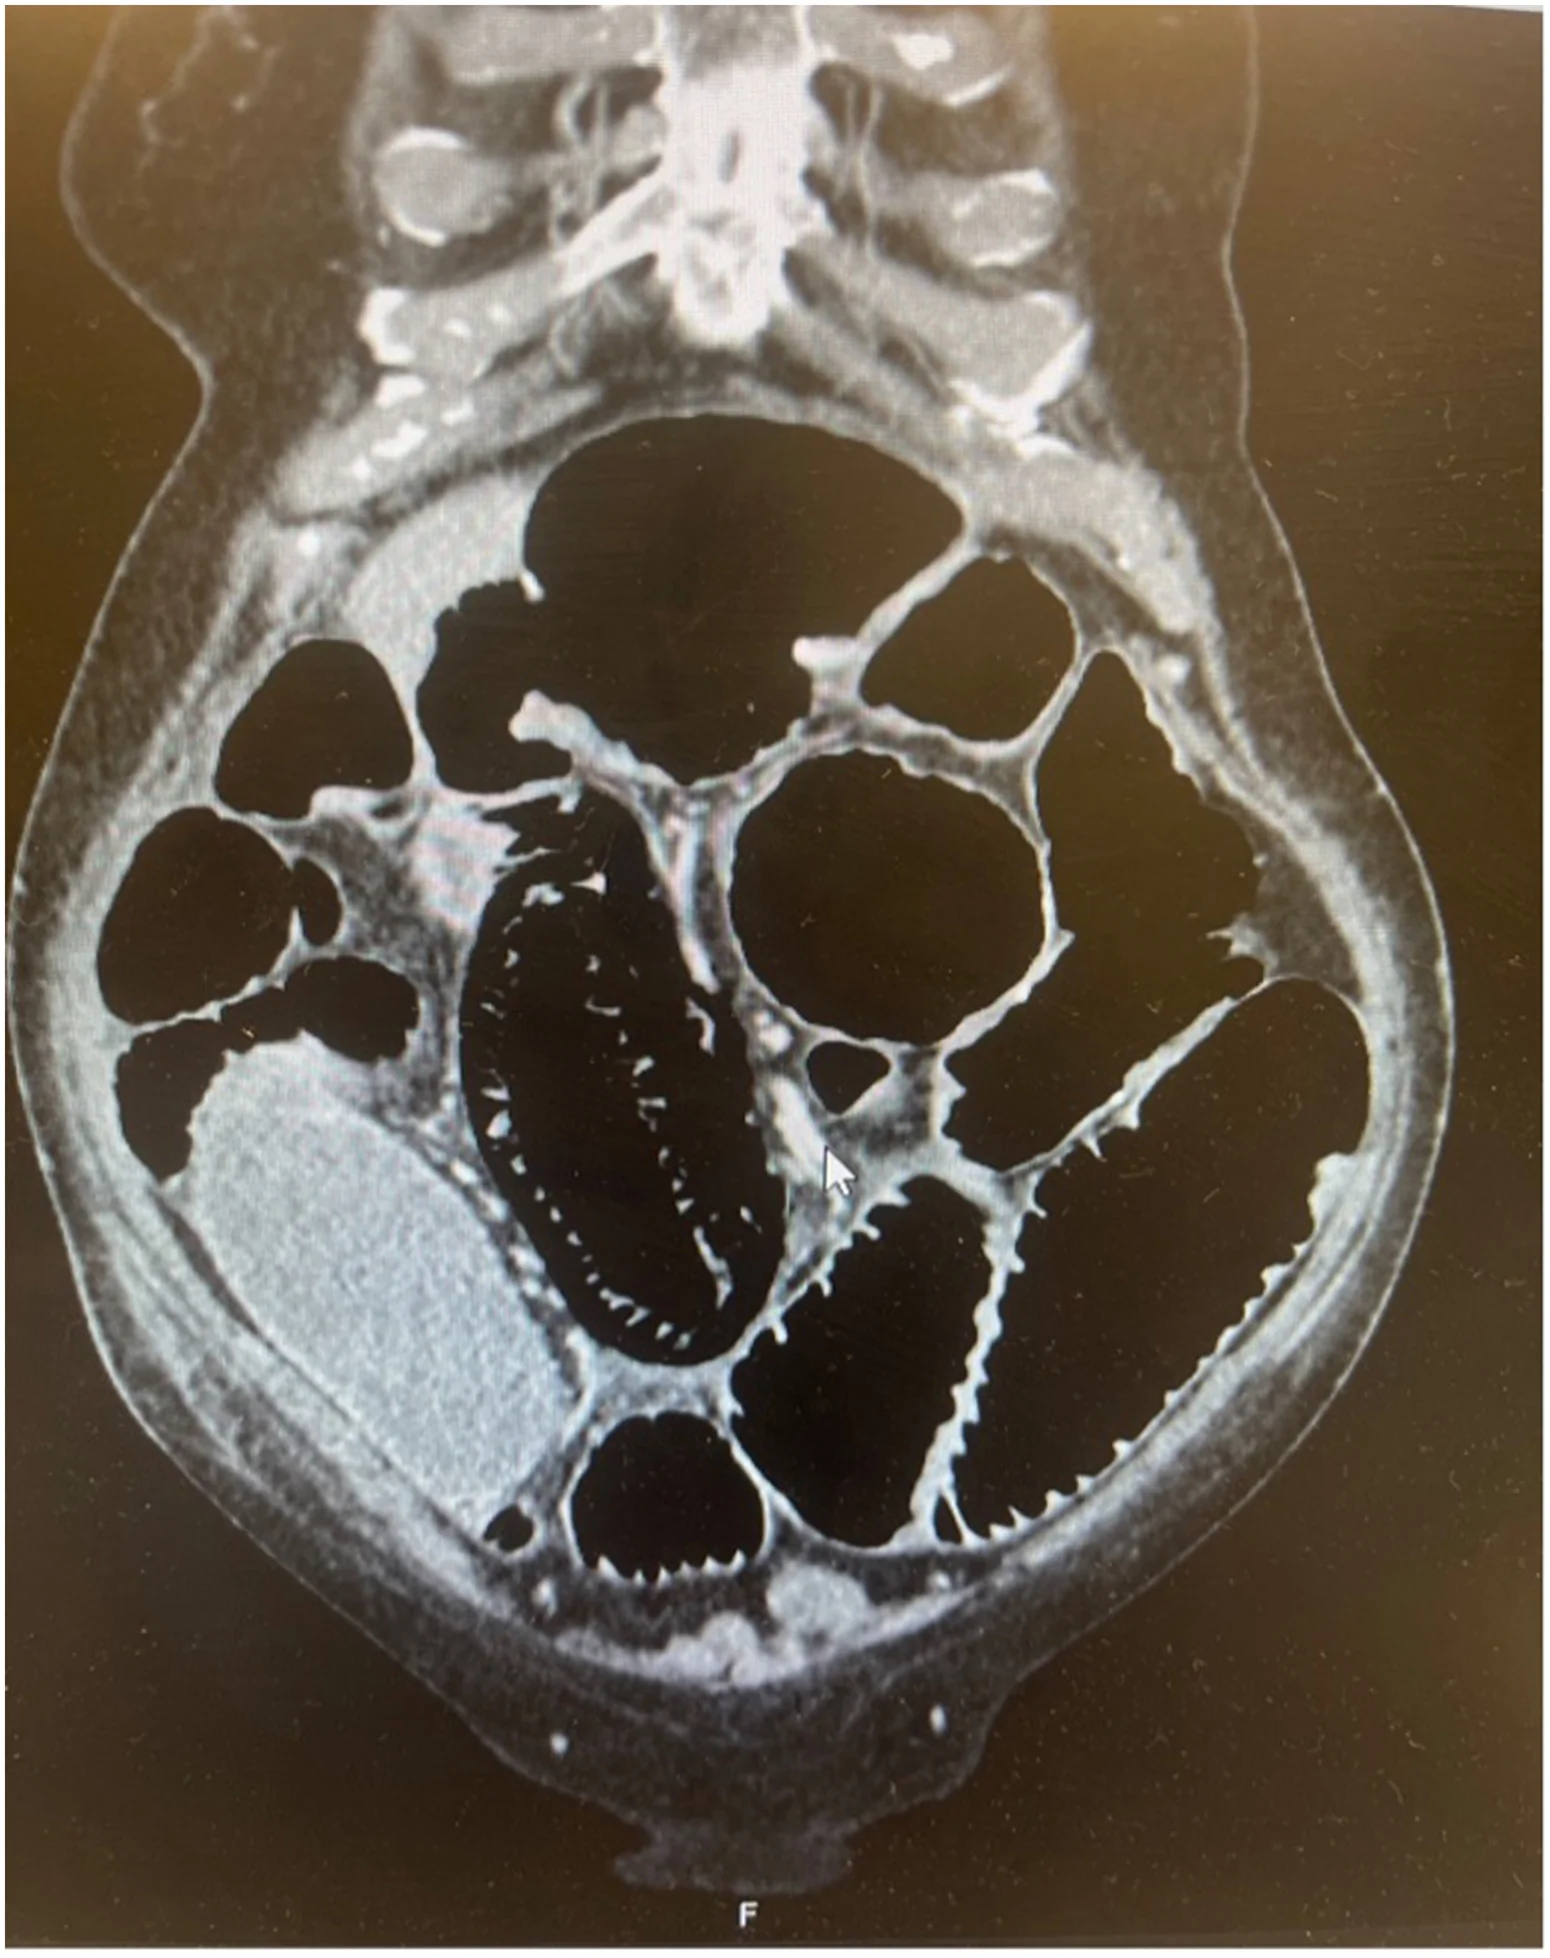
Coronal CT image (POD seven) demonstrating segmental small bowel PI. This coronal section shows multiple loops of dilated small bowel with intramural gas noted along a 20cm segment of the jejunum, distal to the gastrojejunostomy. The pneumatosis appears as small gas collections tracking within the bowel wall, raising concern for possible ischaemia. No pneumoperitoneum or portal venous gas is seen. CT = computed tomography; PI = pneumatosis intestinalis; POD = postoperative day
